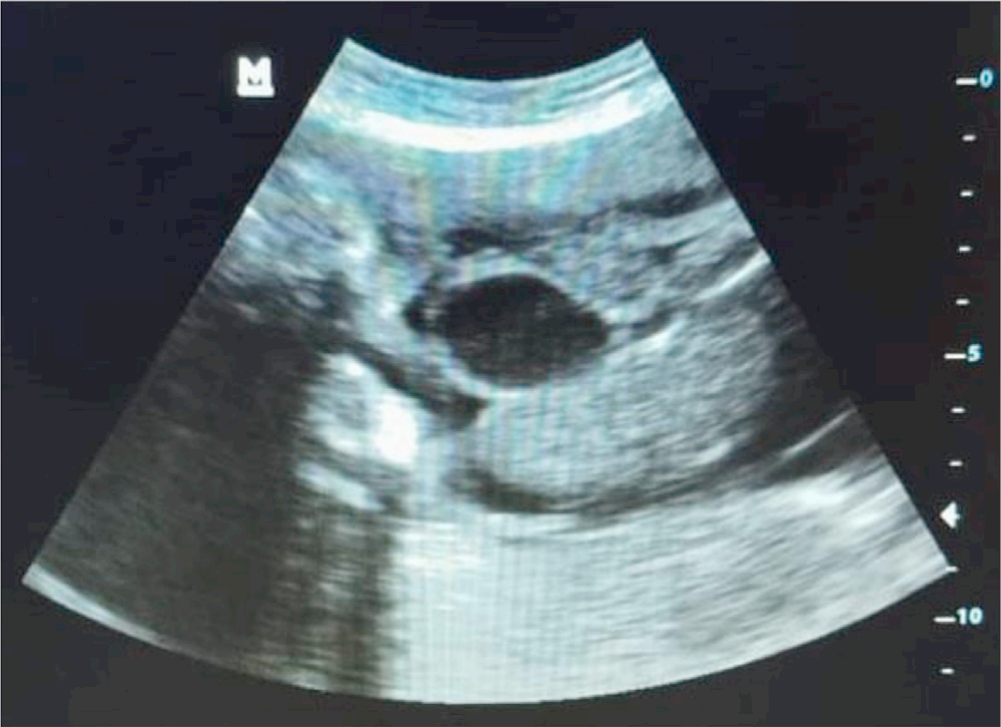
Prenatal ultrasound at 28 weeks gestation (transverse view of fetal abdomen). Extruded abdominal contents are visible anterior to the fetal abdomen, with some areas suggesting partial encasement by thin membranous structures.
